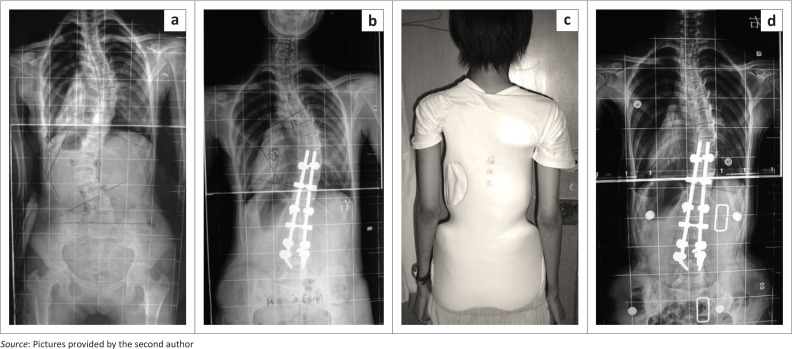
(a) X-ray of a patient with a Lenke 3C pattern before undergoing surgery. (b) Only the lumbar part was fused, and because of the decompensation of the thoracic curve, a brace (c) was made with moderate correction of the thoracic curve (d). With appropriate planning of this surgery, these issues would have been avoidable.
